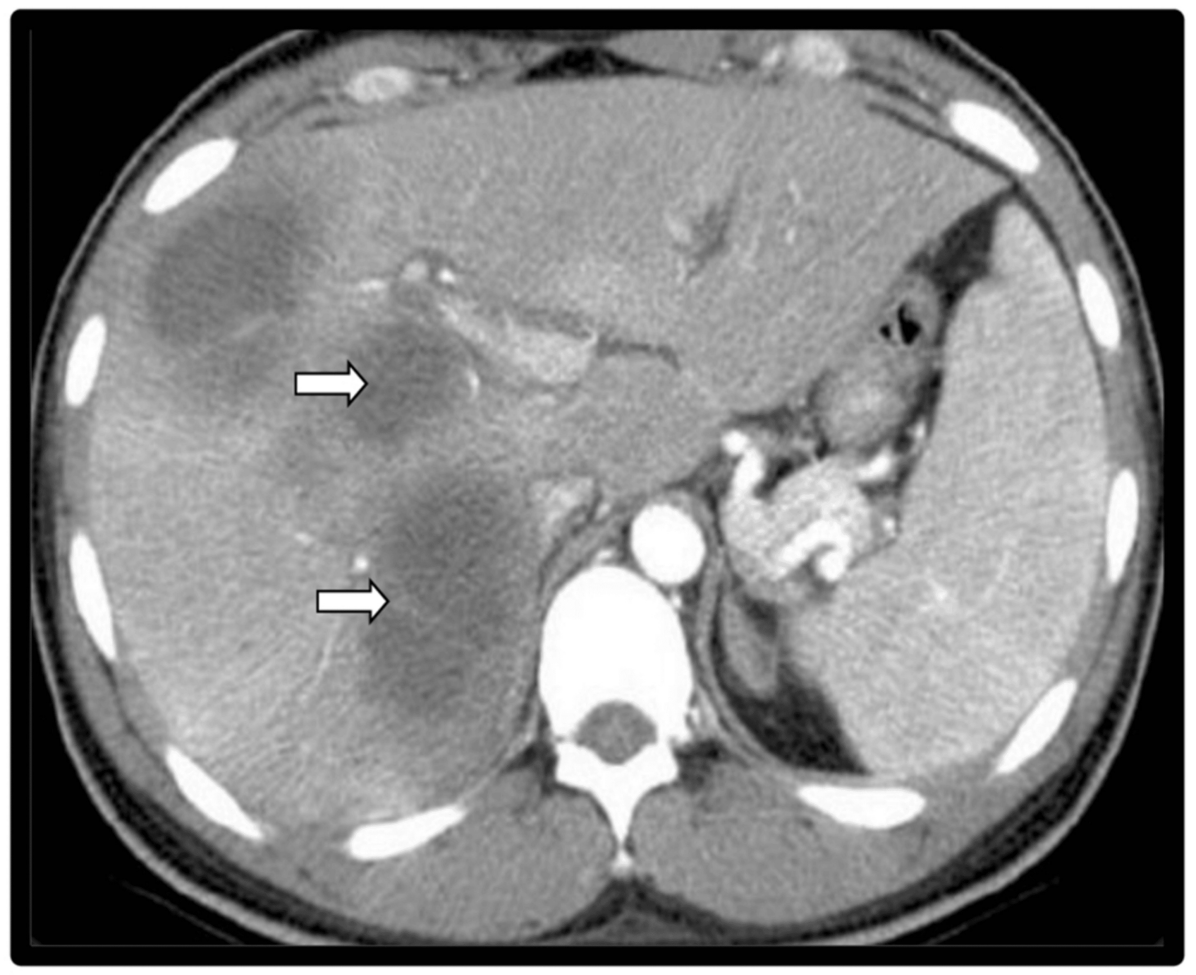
Contrast-enhanced computed tomography (CECT) (abdomen) image showing multiple liver abscesses
